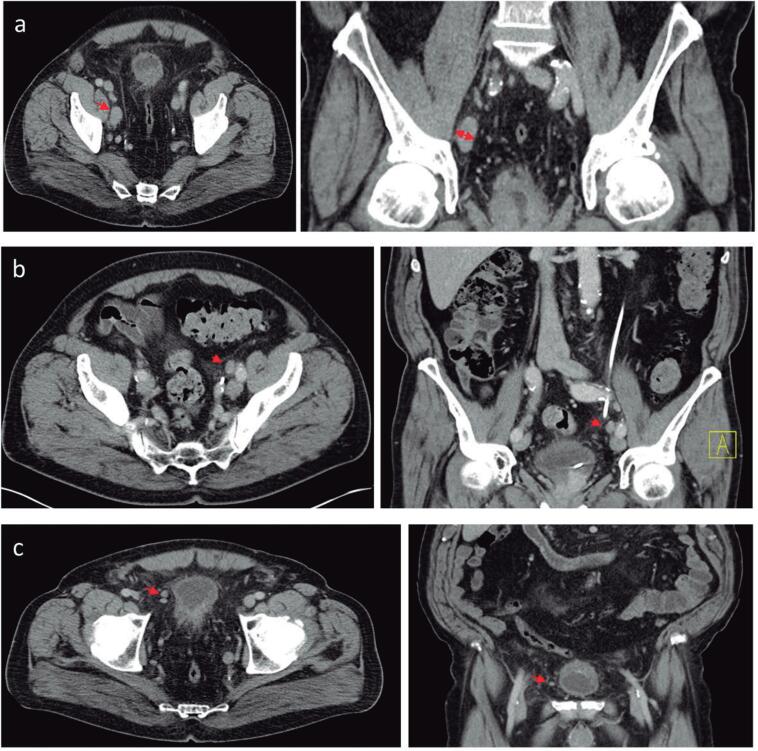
Morphological characteristics of lymph nodes in CT scan Representative pelvic CT scan showing lymph node characteristics such as size (A), morphology (B) and loco-regional number (C) in transversal and coronal plane.
